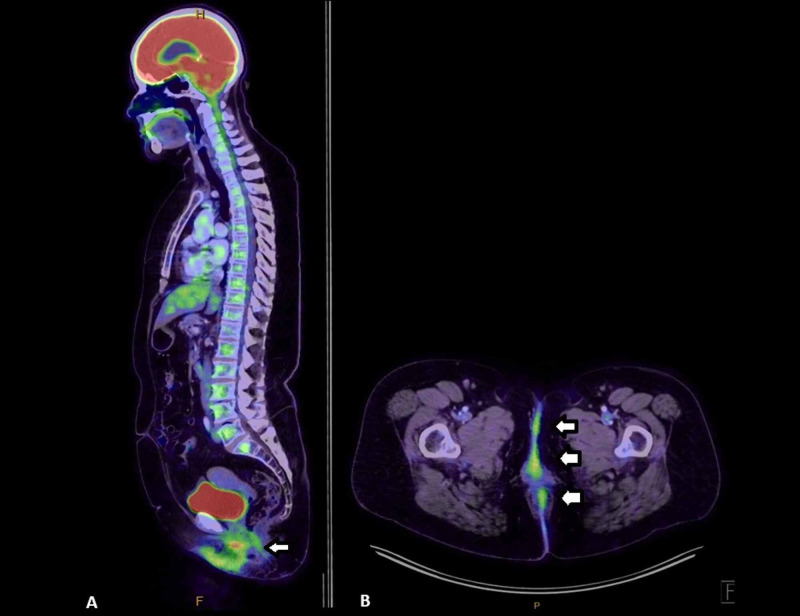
Varying degrees of increased FDG activity around the perianal region, natal cleft, and anterior perineum (white arrows) on PET imaging A) sagittal plane. B) axial plane PET: positron emission tomography
